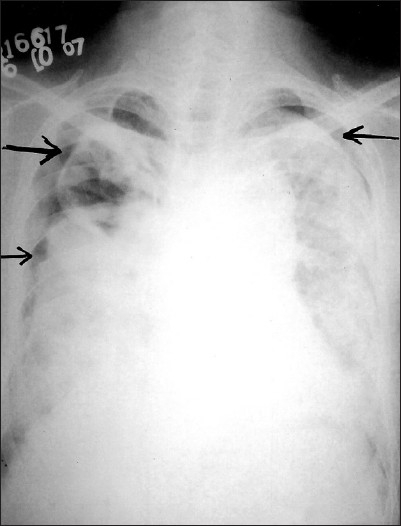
Chest radiograph (PA view) showing bilateral pneumothorax (arrows) with diffuse reticulonodular shadows)
